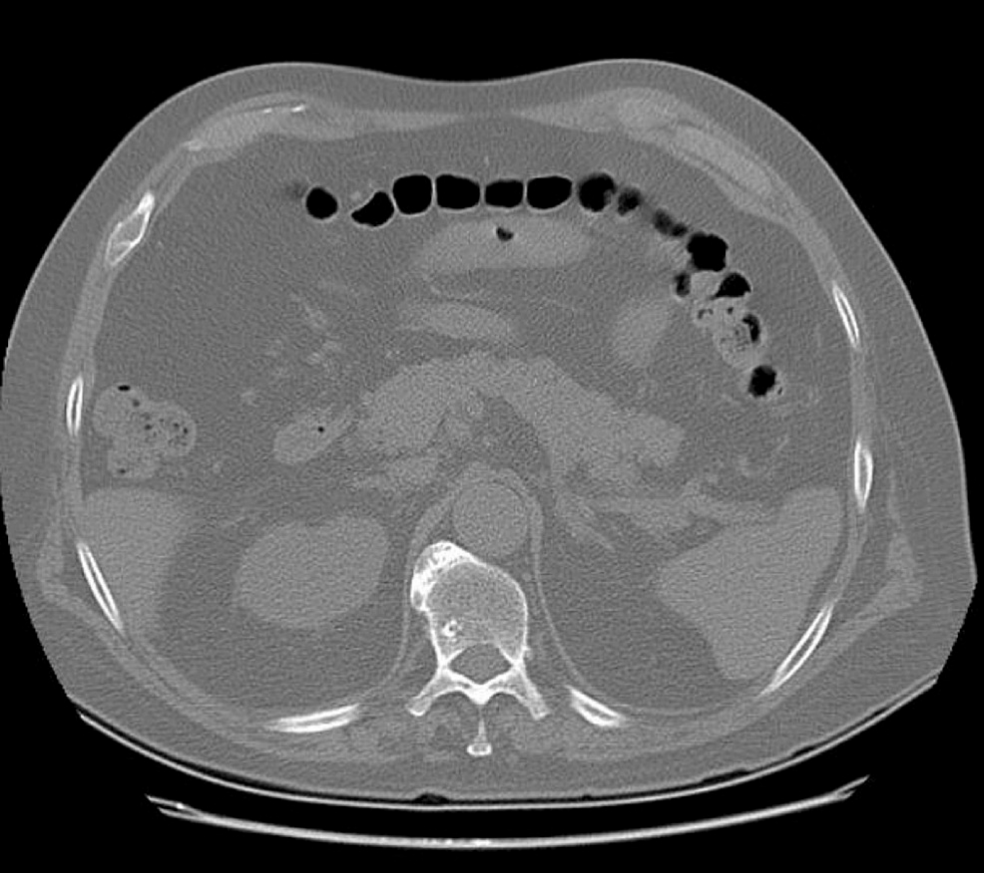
Admission CT of the abdomen without contrast showing no cystic lesions or masses
